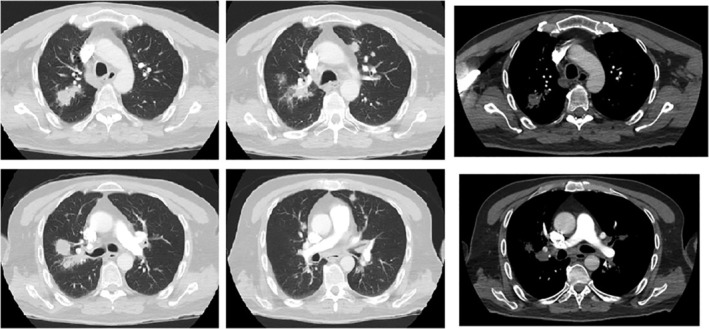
Computed tomography pulmonary angiogram showing two right upper lobe spiculated lung masses with multiple bilateral pulmonary nodules and mediastinal lymphadenopathy
